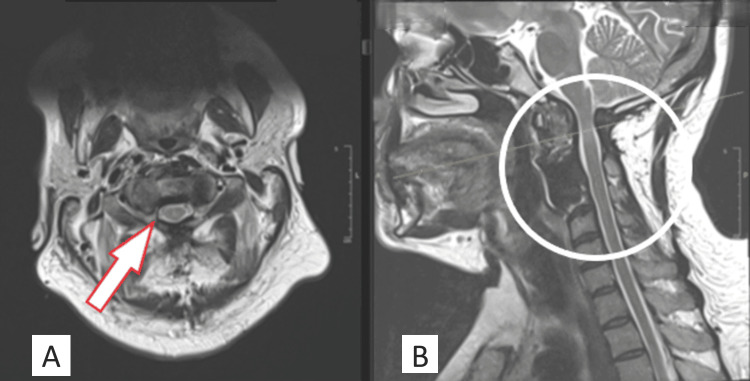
MRI done six months post-operative. The axial view (A) showed improvement of the cord compression with CSF all around the cord and full excision of the mass (arrow). The sagittal view (B) showed the hypodensity signal at the site of C3 corpectomy, with no sign of collection, adequate spinal cord decompression, and improvement of intramedullary signal hyperintensity of the spinal cord (circle). CSF: cerebrospinal fluid
